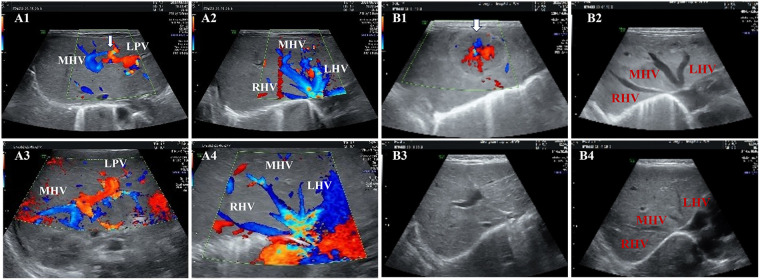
The hepatic ultrasound image of two congenital portosystemic shunt cases. (A1–4) Case 1—serial changes on hepatic ultrasound images. (A1) The arrow indicates the left portal vein branch with communication to the middle hepatic vein; (A2) hepatic vein dilatation secondary to the shunt (at admission); (A3) no visible communication between the portal vein and the hepatic vein (at 1-month follow-up); (A4) reduction in the caliber of the hepatic vein after the closure of the shunt. (B1–4) Case 2—serial changes on hepatic ultrasound images. (B1) The arrow indicates a portosystemic shunt; (B2) hepatic vein dilatation secondary to portosystemic shunting, and the degree of shunt flow is proportional to the number of hyperechoic foci (at admission); (B3) the hyperechoic foci resolved after the closure of the shunt (at 7-month follow-up); (B4) reduction in the caliber of the hepatic vein after the closure of the shunt. PV, portal vein; LPV, left portal vein; LHV, left hepatic vein; LRV, left renal vein; MHV, middle hepatic vein; RPV, right portal vein; RHV, right hepatic vein; SV, splenic vein; UV, umbilical vein.
